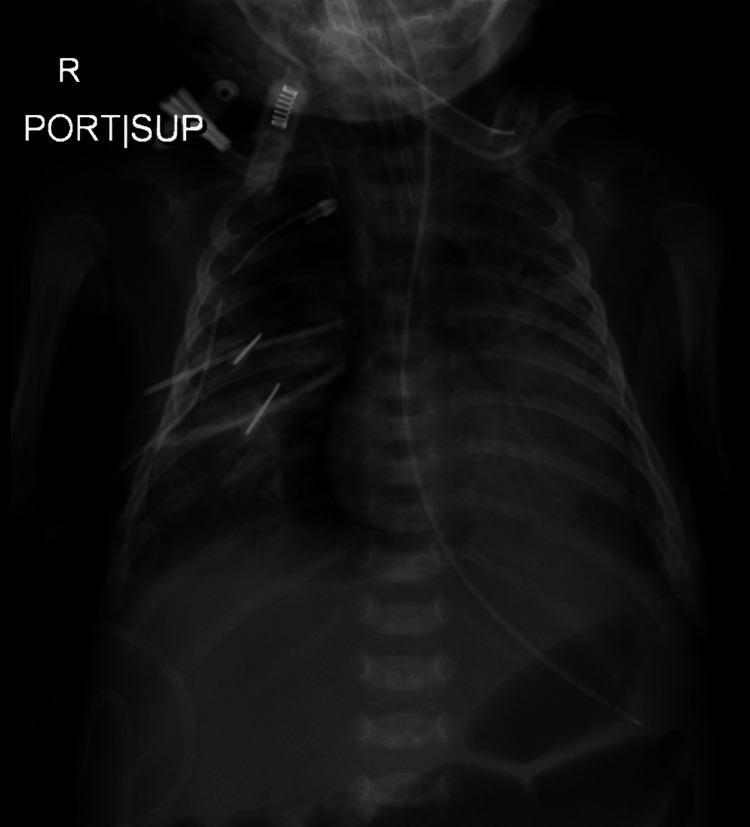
Chest X-ray (anteroposterior view) on the day of death showing pneumomediastinum and bilateral diffuse airspace shadowing.
